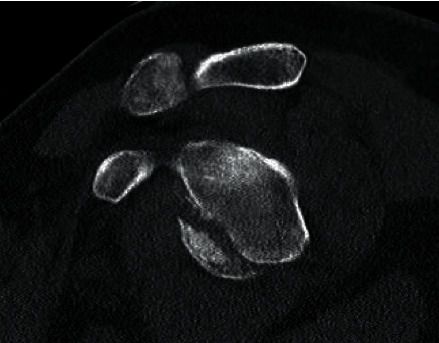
Sagittal CT image demonstrating large anteroinferior glenoid rim fracture involving approximately 25% of the glenoid width.
